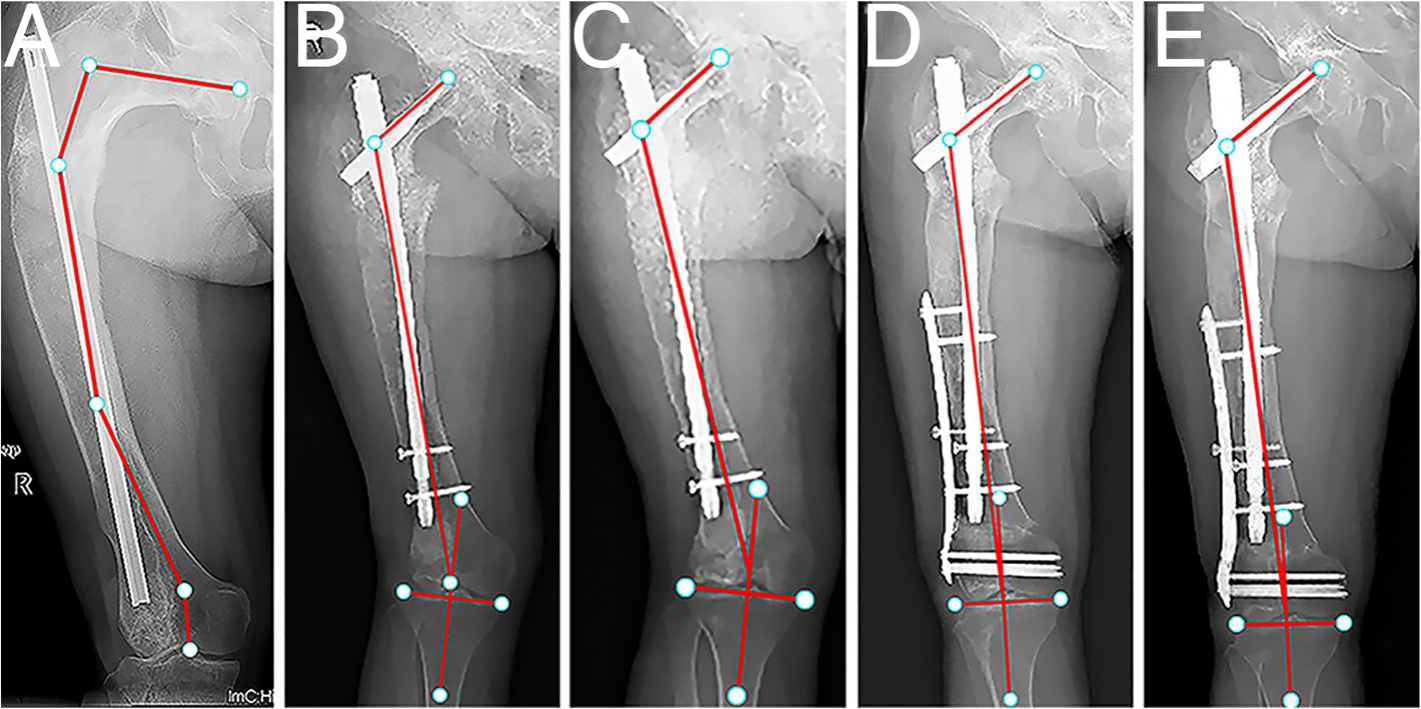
a Type 5 lesion in a 36-year-old woman. The entire femur was affected by fibrous dysplasia. The neck-shaft angle was 97°. Coxa vara, varus deformity in the proximal femoral shaft, and juxtaarticular valgus deformity in the distal femur were all present, along with knee osteoarthritis. b The coxa vara and varus deformity were corrected with single-level valgus osteotomy made at the vertex of the deformity. The neck-shaft angle was restored to normal (120°). A secondary genu valgum was present with a 20° valgus femorotibial angle. c The osteotomy healed 6 months after the operation. d A supracondylar femoral varus osteotomy was performed to correct the secondary genu valgum. The femur alignment was restored to normal. e A radiograph 96 months after the supracondylar femoral varus osteotomy showed no recurrent deformity
